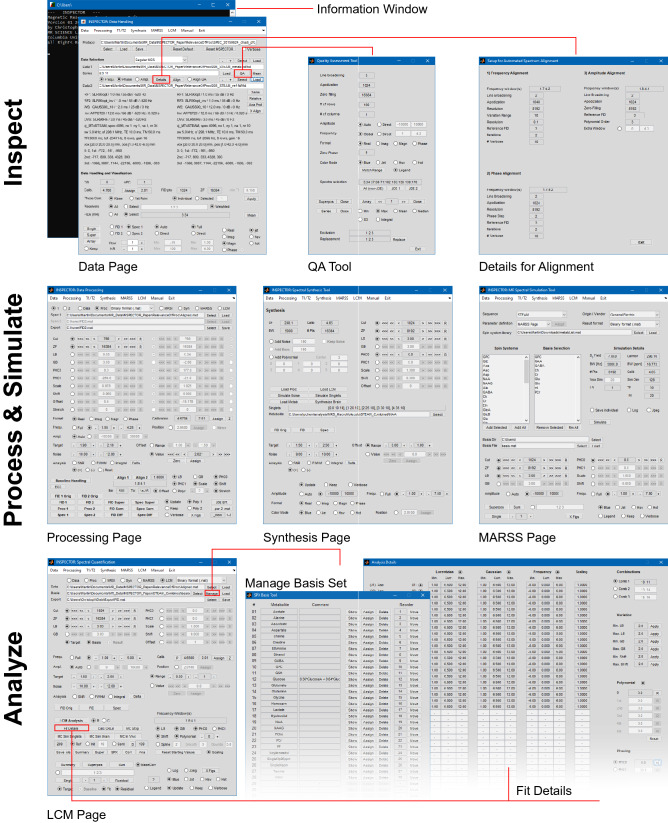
Graphical user interface in INSPECTOR for Microsoft Windows 10. The pages can be selected on the menu bar, and data can be transferred between pages.
